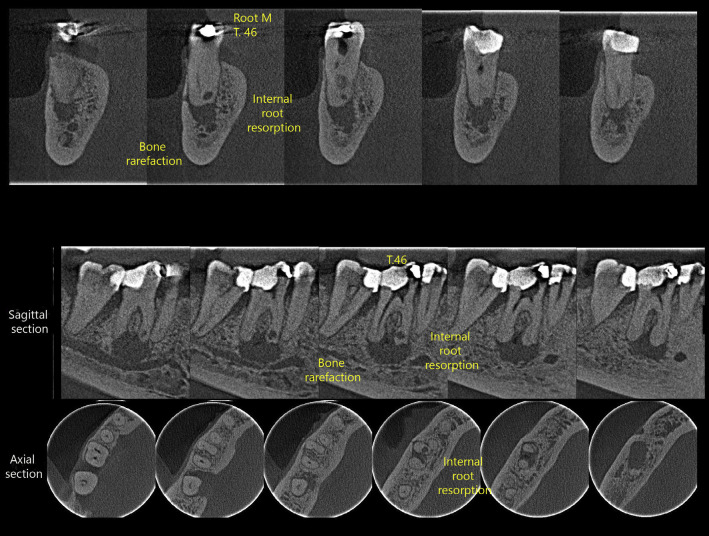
Computed tomography as an imaging test for the differential diagnosis of internal root resorption.
